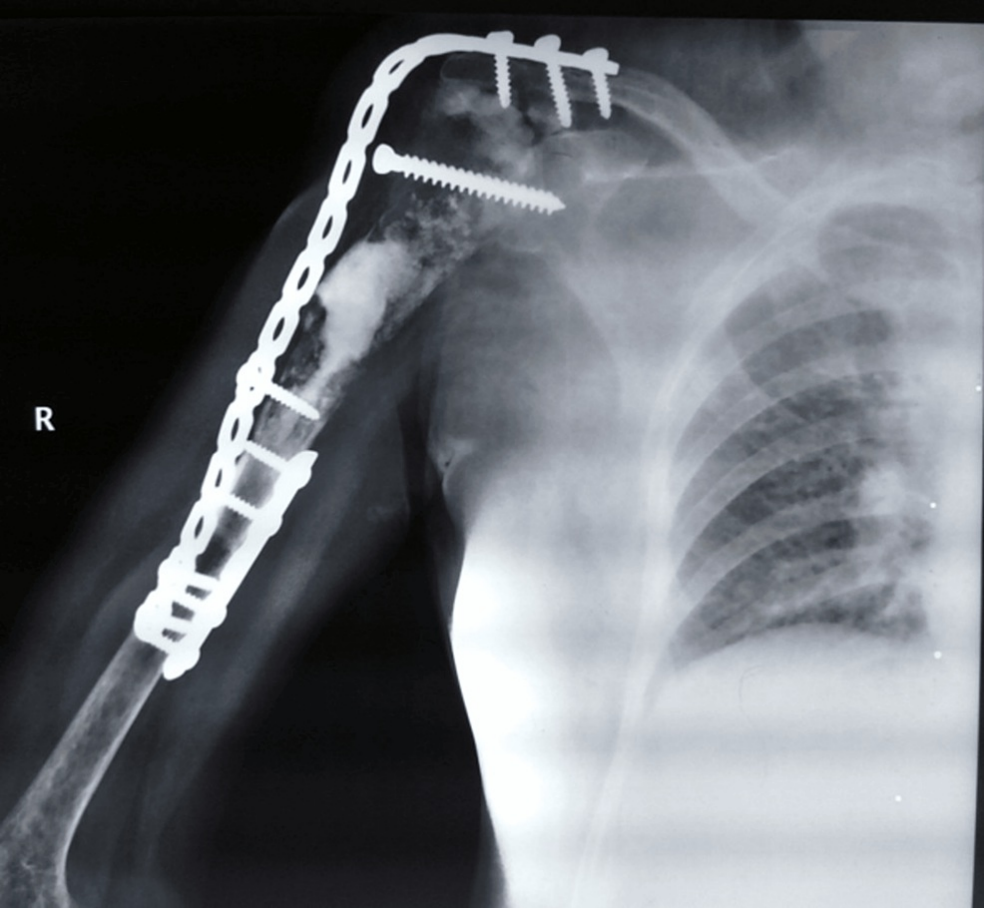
Radiographs at a one-year follow-up
